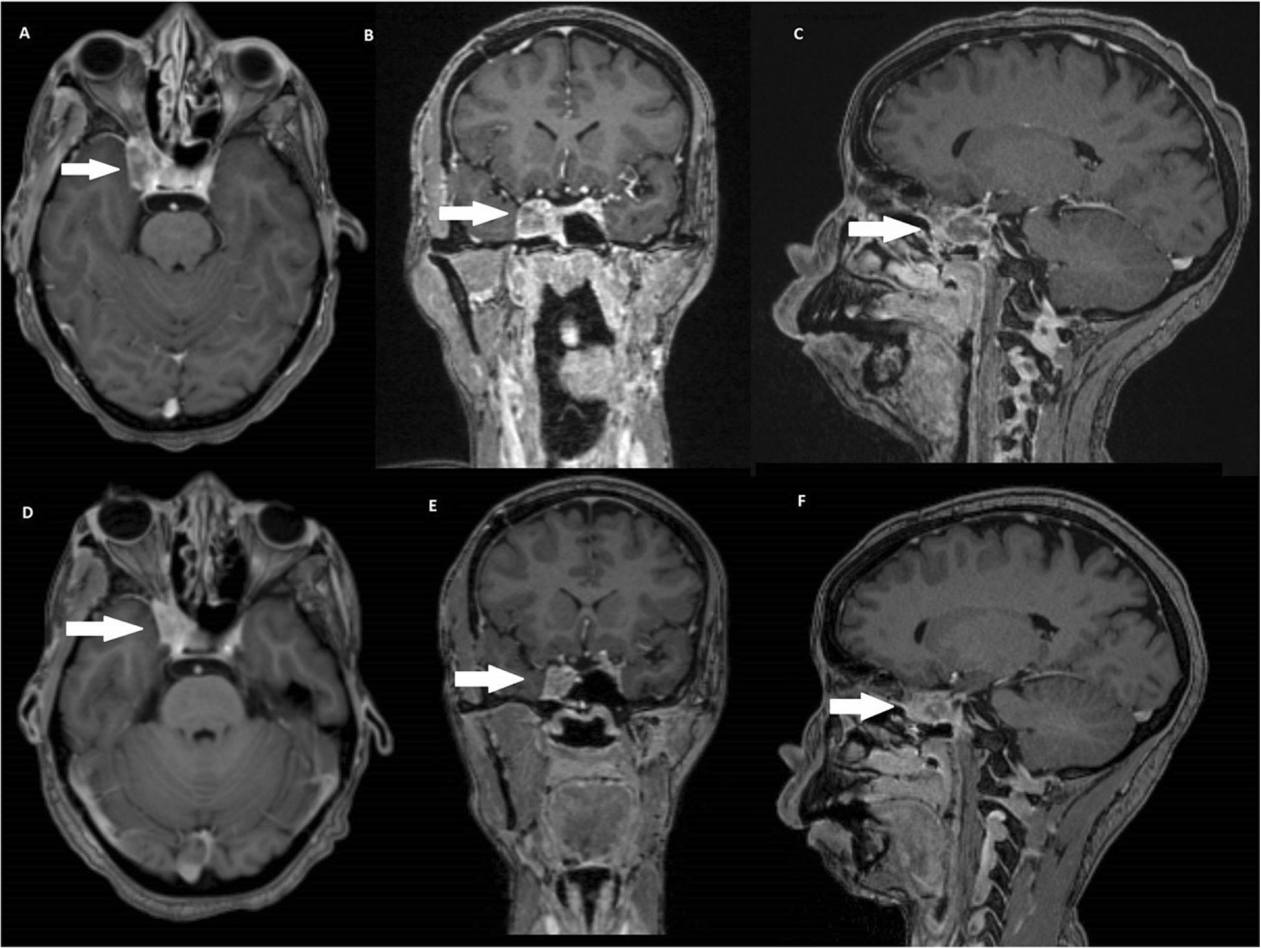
Images a, b, and c: Contrast-enhanced T1-weighted magnetic ressonance imaging (MRI) acquired prior to initiation of treatment (September, 2016), demonstrating lesion with heterogeneous enhancement (arrows) in the right cavernous sinus with insinuation in the superior orbital fissure measuring 24 × 21 × 19 mm. Images d, e and +: Brain MRI one year after the initiation of Vemurafenib (September 2017) showing significant reduction of lesion dimensions, measuring 18 × 13 × 14 mm
